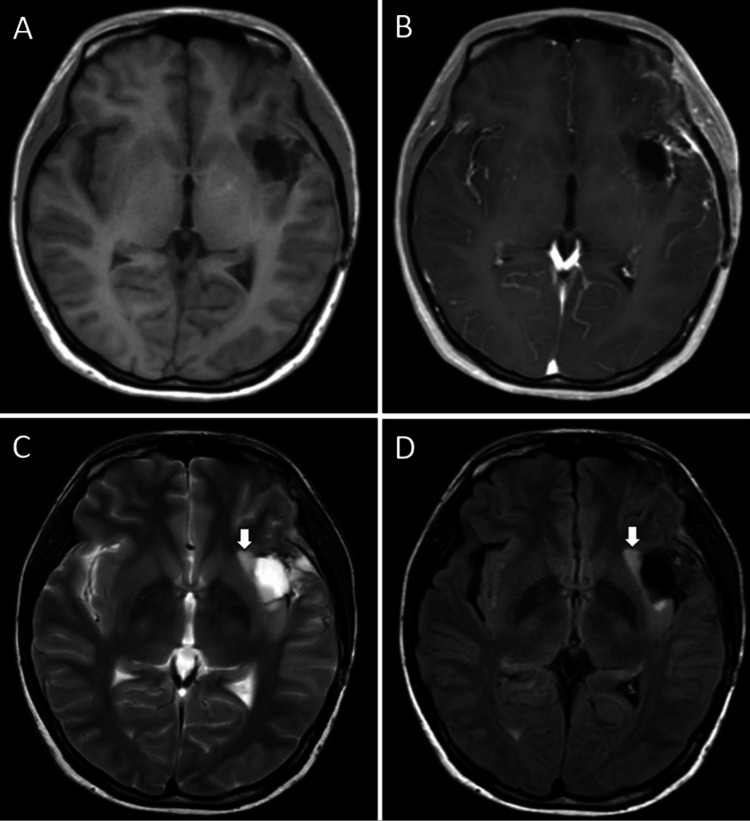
Postoperative and post-chemoradiation MRI demonstrating residual diffuse insular glioma. The non-contrast T1-weighted image (A) and contrast-enhanced T1-weighted image (B) show removal of the previously enhancing rim. The T2-weighted image (C) and FLAIR image (D) demonstrate residual tumor (arrows).
